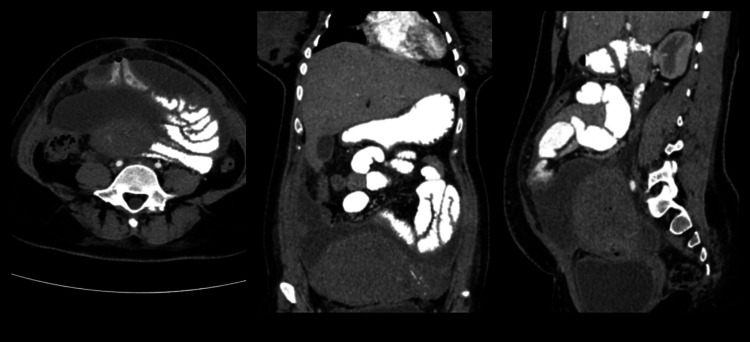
CT scan showing intra-abdominal fluid-filled lesion in the lower abdomen, anterior to the uterus and beneath the abdominal wall.
